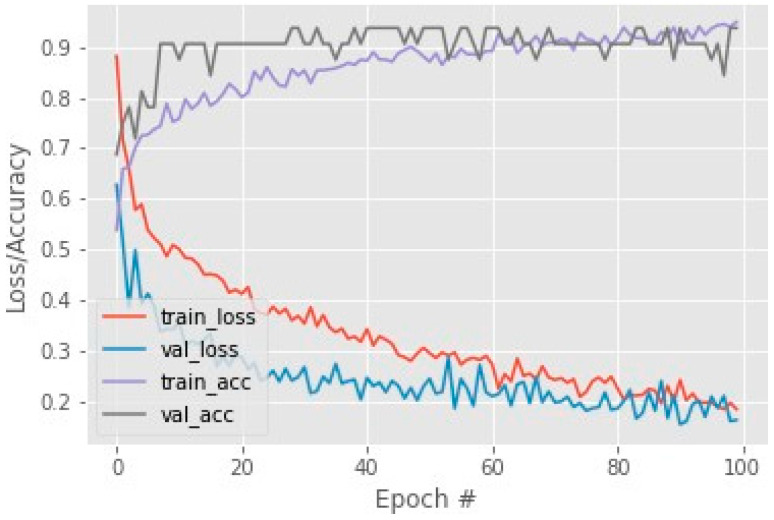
COVID-CT dataset training loss and accuracy.
